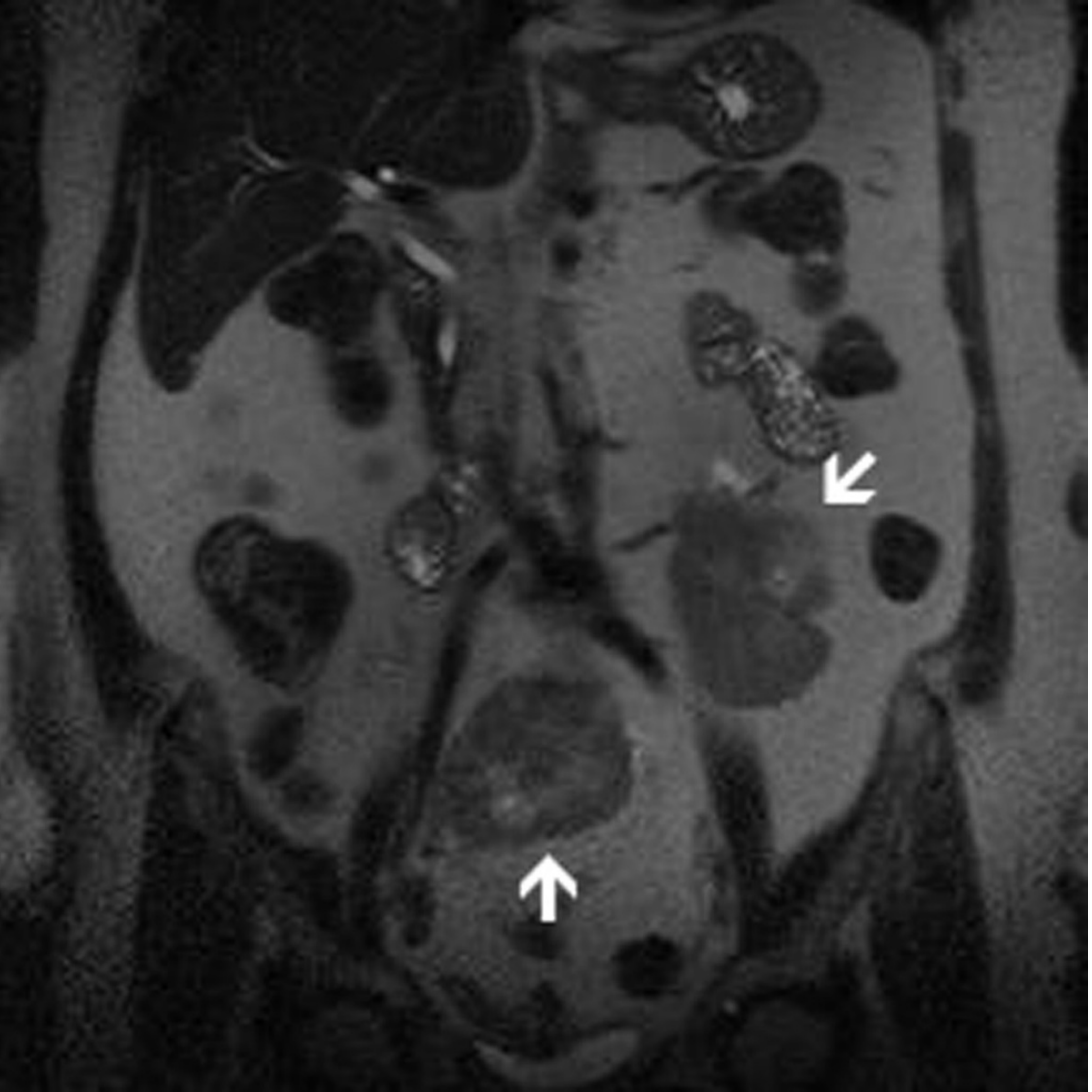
T2-weighted half Fourier single-shot turbo spin echo (HASTE) sequence showing bilateral renal ectopy (arrows).
